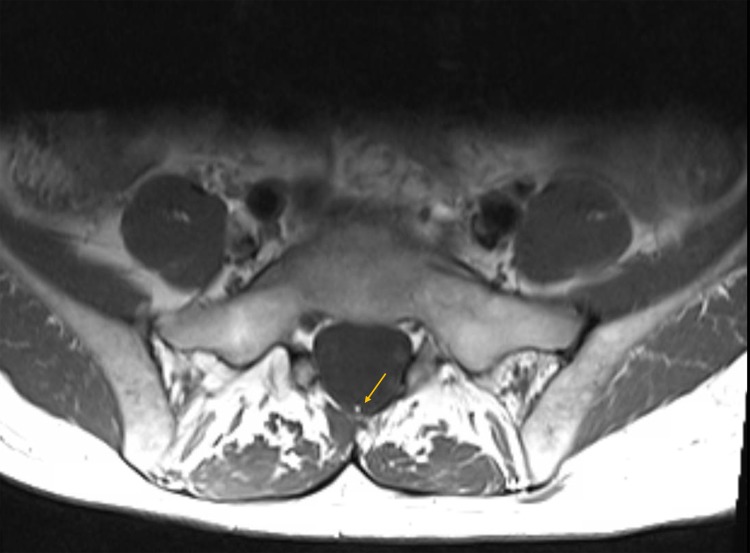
Axial T1 magnetic resonance imaging scan at the level of the L5 vertebral body showing a fatty filum (yellow arrow)
